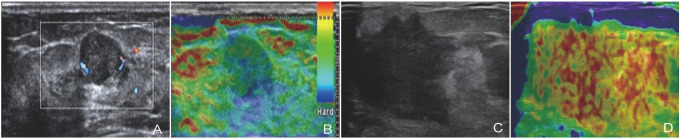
Conventional ultrasound and UE representative images of breast lesions. (A and B) Conventional ultrasound and UE images of benign breast lesions; (C and D) Conventional ultrasound and UE images of malignant breast lesions. UE: Ultrasound elastography.
